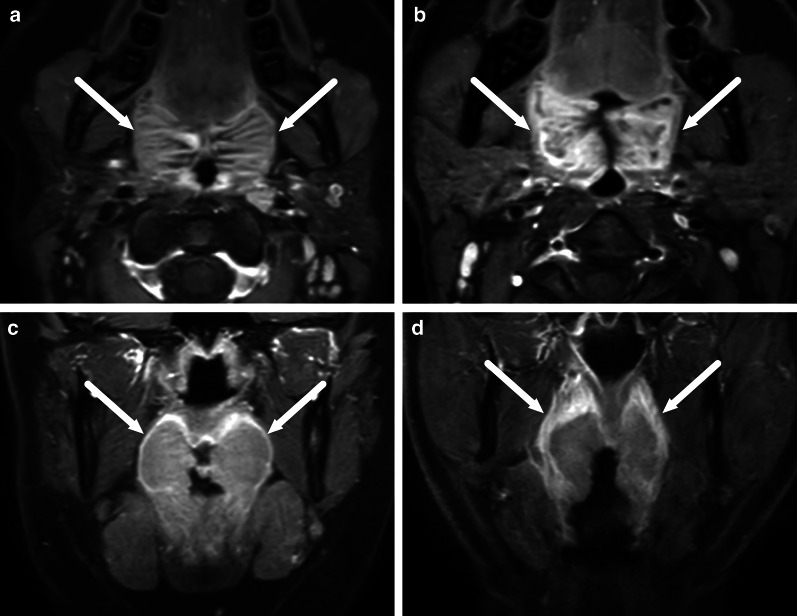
Tonsillitis/peritonsillitis on axial (a, b) and coronal (c, d) post-contrast T1-weighted images in two patients (a and c, b and d). Note the striped appearance of the tonsils (arrows in a), peritonsillar enhancement (arrows in b–d), and no abscess
